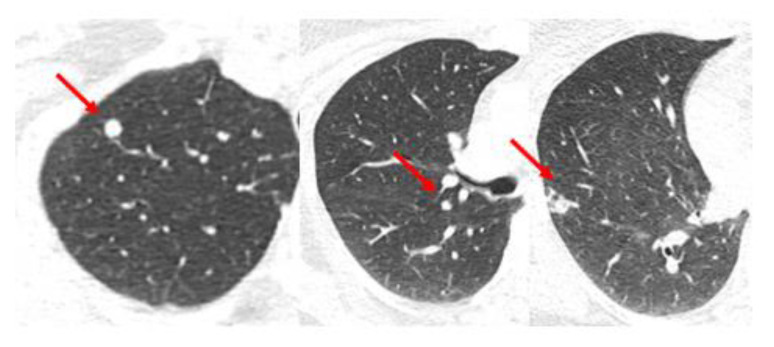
Case 2: Simultaneous oligometastases. Three representative axial images from a CT scan obtained for initial staging of breast cancer showed 3 highly suspicious lung nodules each marked with a red arrow that were PET-avid. With systemic therapy alone there has been a complete response in the lung nodules for greater than 3 years.
